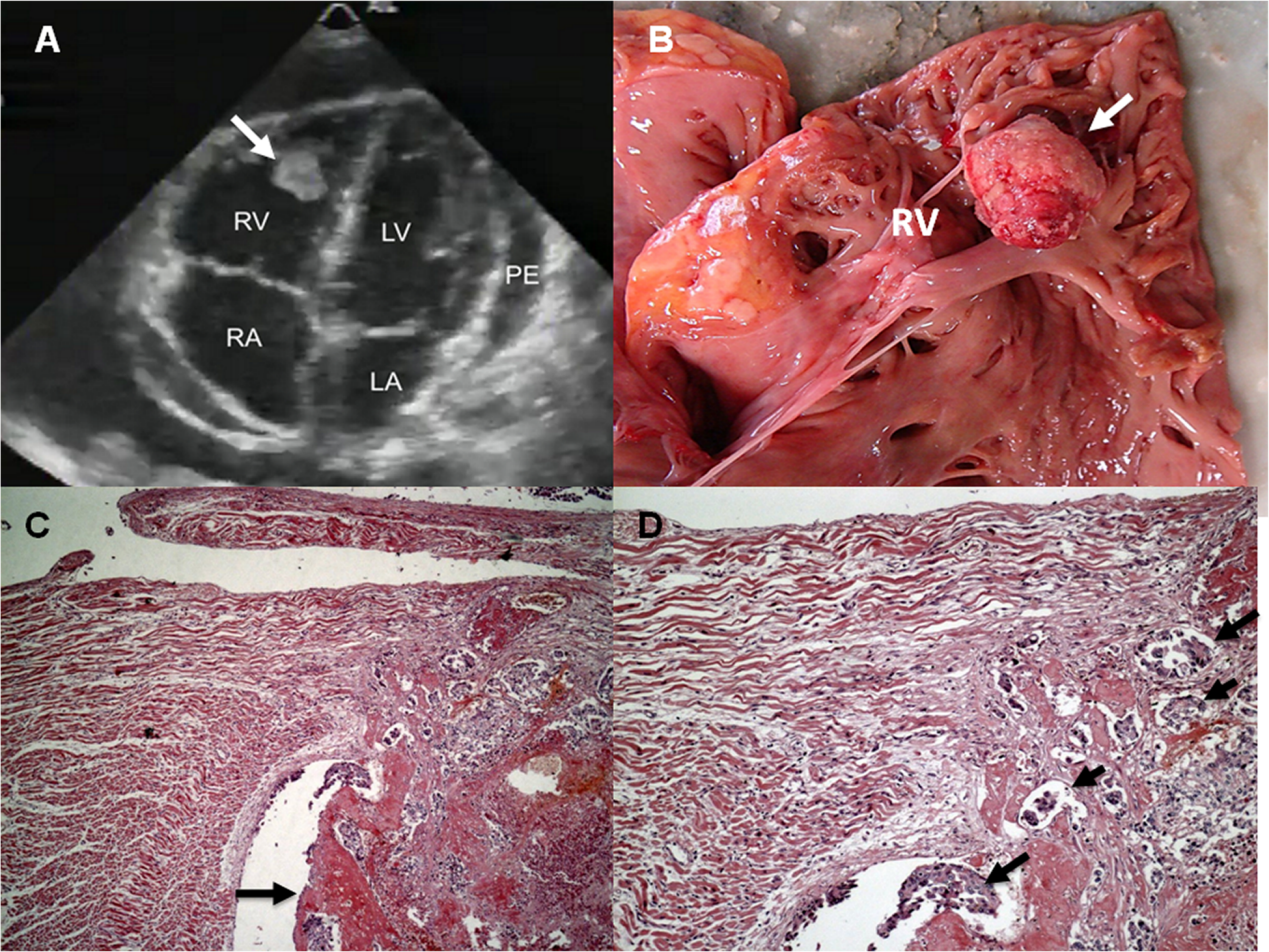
Cardiac involvement by the tumor. A: Image from transthoracic echocardiogram showing a mass attached to the apex of the right ventricle with a pericardial effusion (white arrow). RV = right ventricle; RA = right atrium; LV = left ventricle; LA = left atrium; PE = pericardial effusion. B: Photography derived from the autopsy showing opened right ventricle with a mass attached to the endocardial surface of the right ventricle (white arrow). RV = right ventricle. C: Polypoid cardiac mass (× 250) (black arrow). D: Emboli of neoplastic cells in myocardial and endocardial vessels (× 400) (black arrows).
